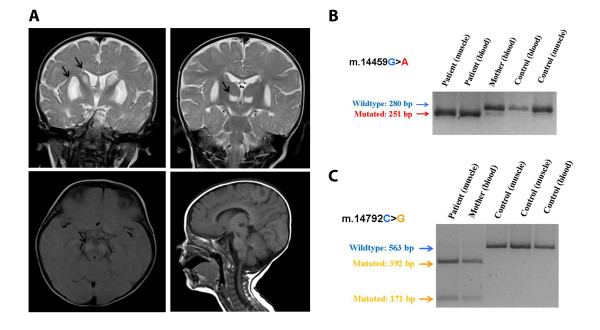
Neuroradiological and molecular features in our proband. A. Brain MRI showed bilateral symmetric hyperintense lesions on T2-weighted images in the head of caudate, putamen (top left), thalami (top right) and ventral mesencephalum (bottom left); these lesions were hypointense in T1-weighted images (bottom right). B. PCR-RFLP analysis of m.14459G > A mutation. The transition m.14459G > A creates a restriction site for endonuclease MaeIII in mutated amplicons obtained using a modified primer set previously described (FOR14430*-RC14710) producing two fragments of 251 and 29 base pairs (the latter is not visible on the agarose gel) while wild type molecules remain uncut. C. PCR-RFLP analysis of m.14792C > G variant. The variant m.14792C > G is recognized by restriction endonuclease HinfI which cuts mutated PCR-amplified fragments (encompassing 14400-14963 nucleotides) producing molecules of 392 and 171 base pairs. Control samples are from healthy unrelated independent subjects.
